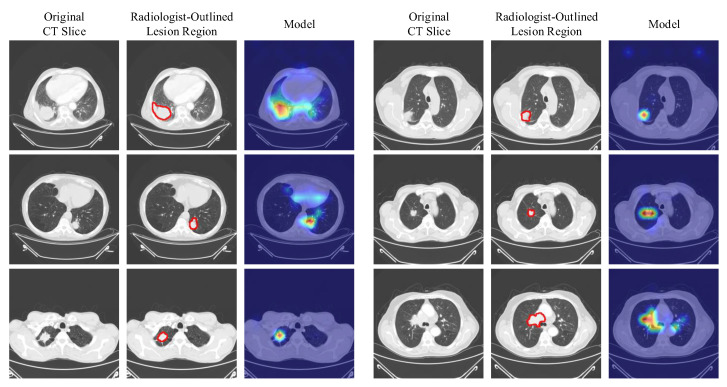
Comparison of radiologist-annotated regions with model-generated attention maps on external CT images. Red contours denote the lesion boundaries manually delineated by the radiologist.
